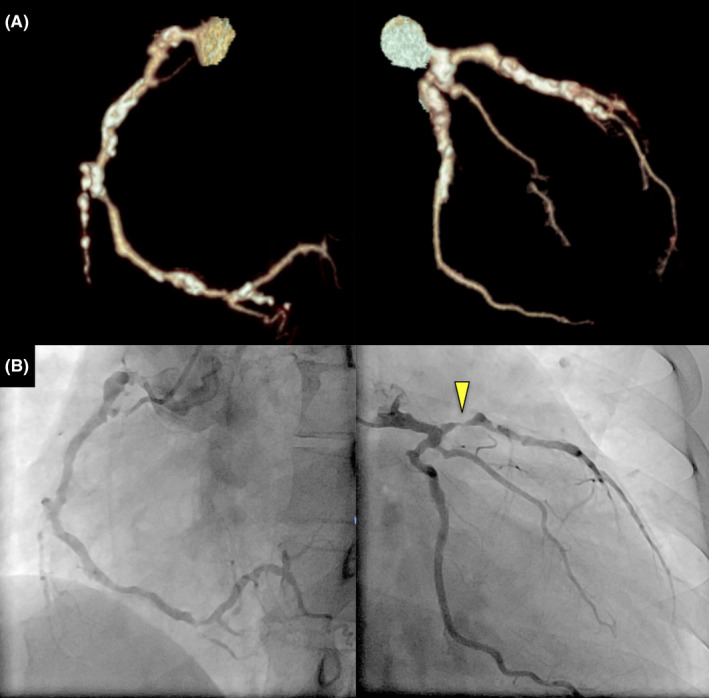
(A) Coronary computed tomography angiography 2 years ago showing diffuse calcifications in the coronary vessels. (B) Present coronary angiography revealing significant stenosis of the proximal left anterior descending artery
